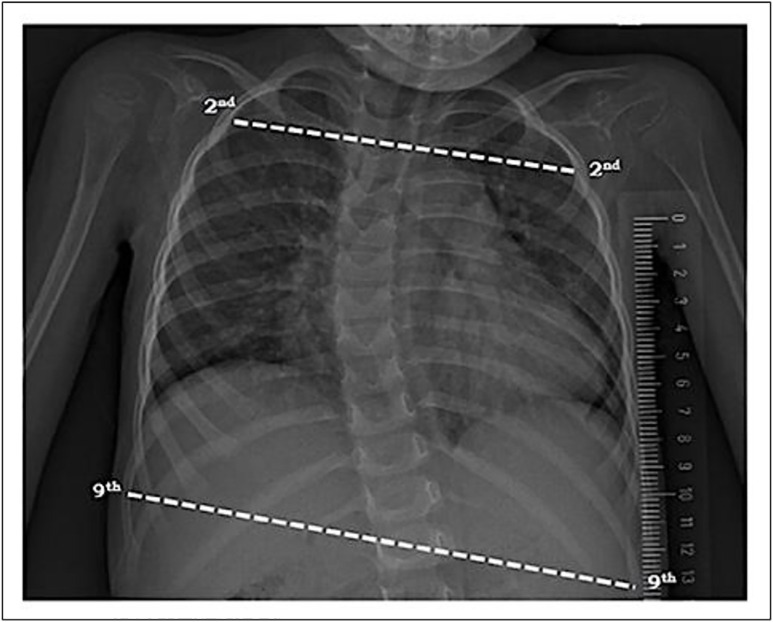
Chest X-ray indicating measurement points for basal upper-lower chest wall ratio.
